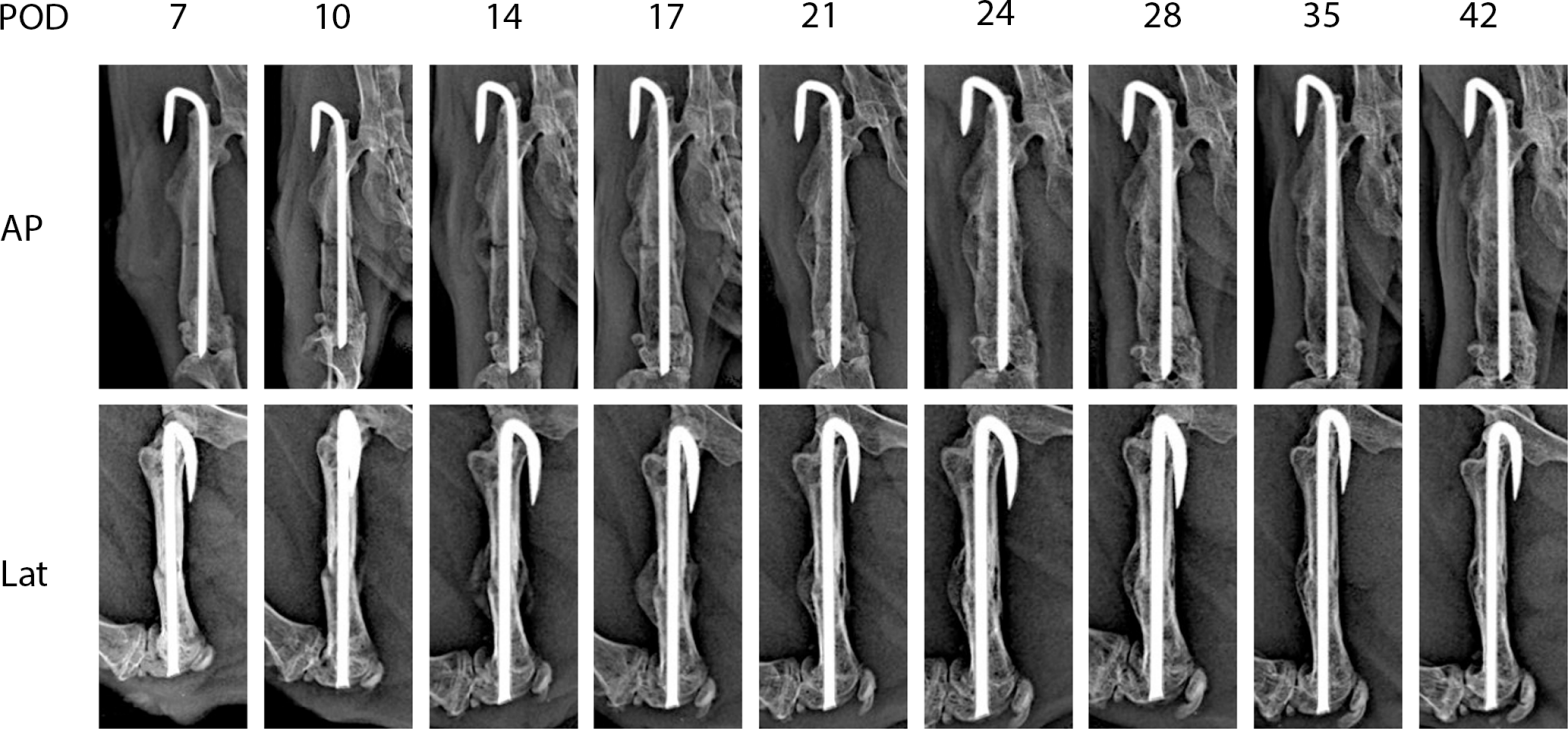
Representative X-rays from each time point used for mRUST scoring. AP, anteroposterior; Lat, lateral; POD, post-operative day.
